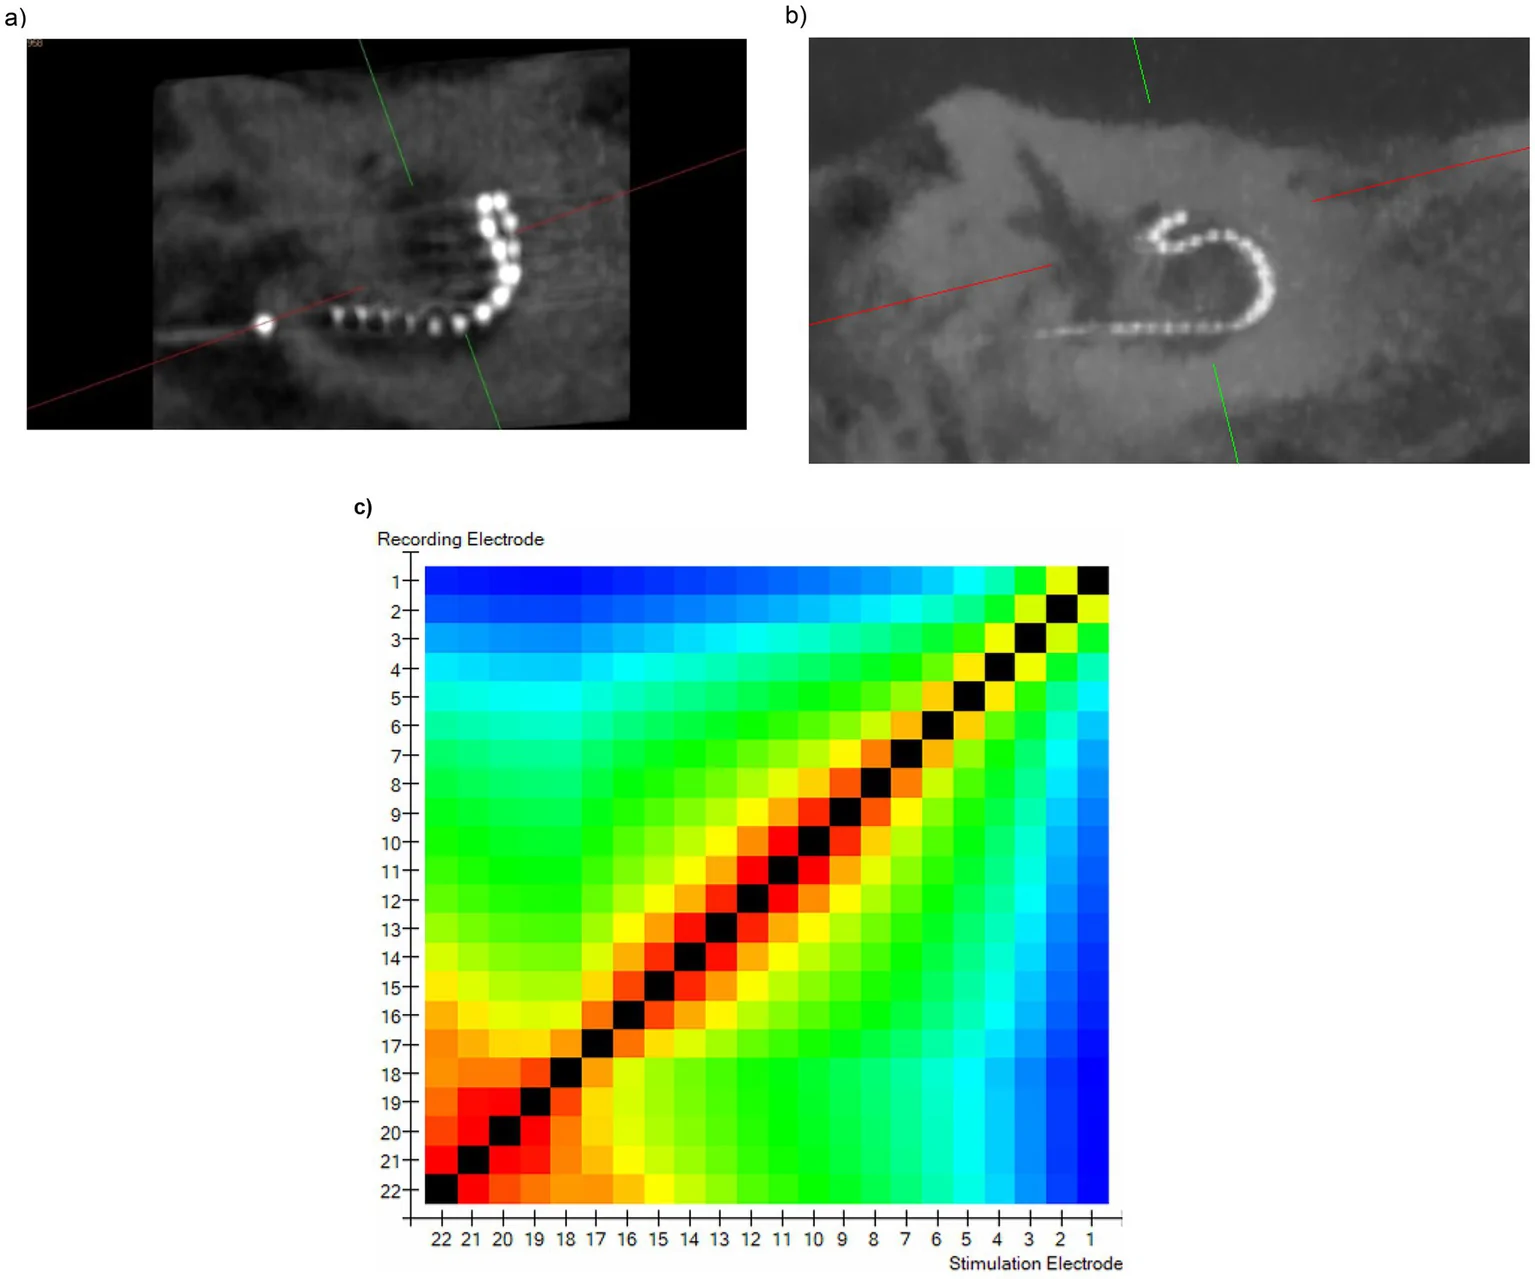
Postoperative cone-beam computed tomography demonstrating electrode array tip fold-over (a) Example of a tip fold-over involving a Mid-Scala (MS) array from Advanced Bionics (b) Example of a tip fold-over involving a Slim Straight Array (SSA) from Cochlear™ (c) Corresponding abnormal intraoperative TIM measurement, which clearly detected the tip fold of the SSA (b).
